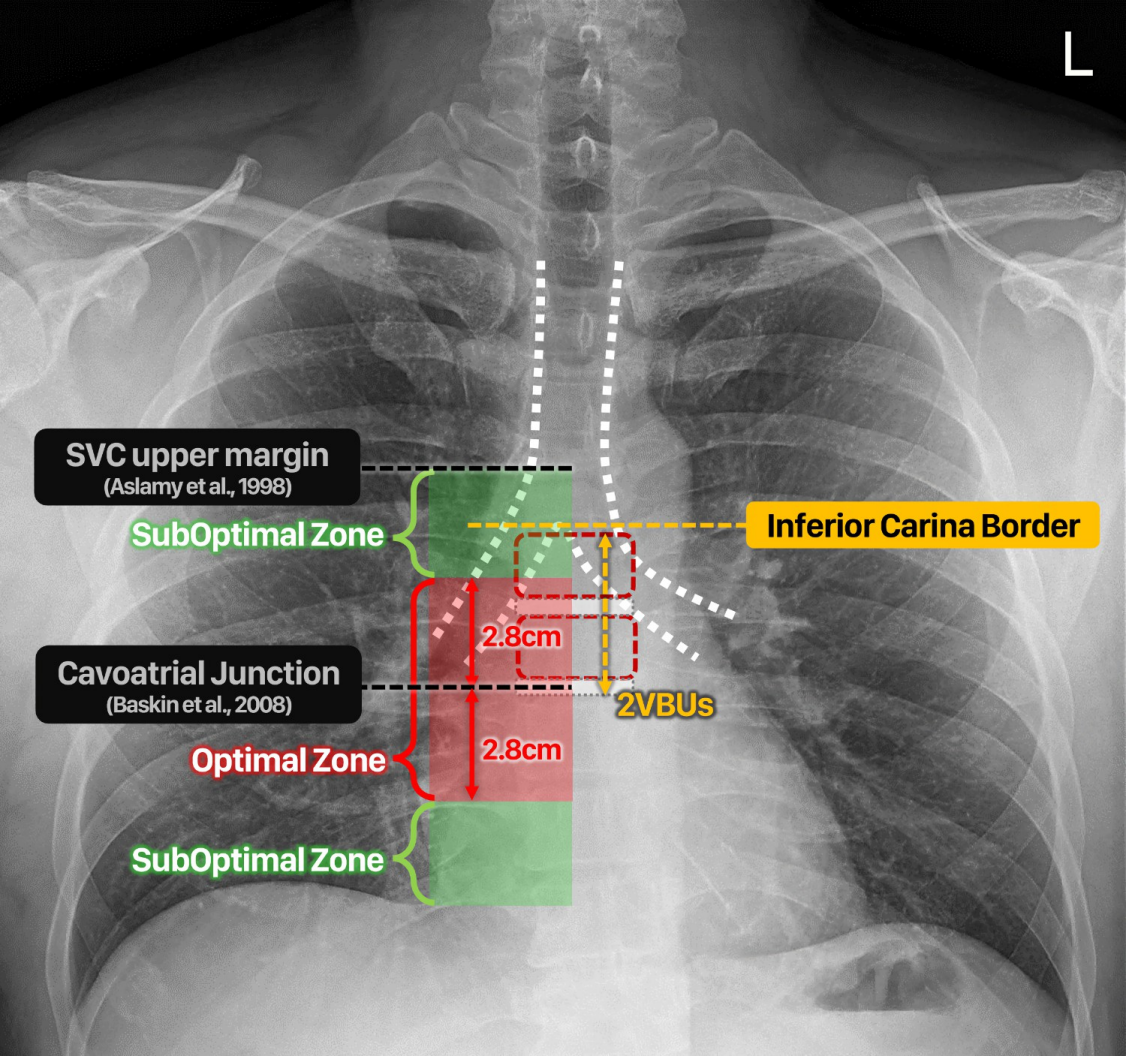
Validation success of catheter length prediction. The results were classified as optimal or suboptimal. In the "optimal" position, the tip of the catheter was located in the range of approximately 2.80 cm above and below the designated cavoatrial junction on anteroposterior chest radiographs. In the "suboptimal" position, the catheter tip was positioned in the superior vena cava (SVC) zone (below the designated upper margin of the SVC) or right atrium without wedging into the atrial wall.
